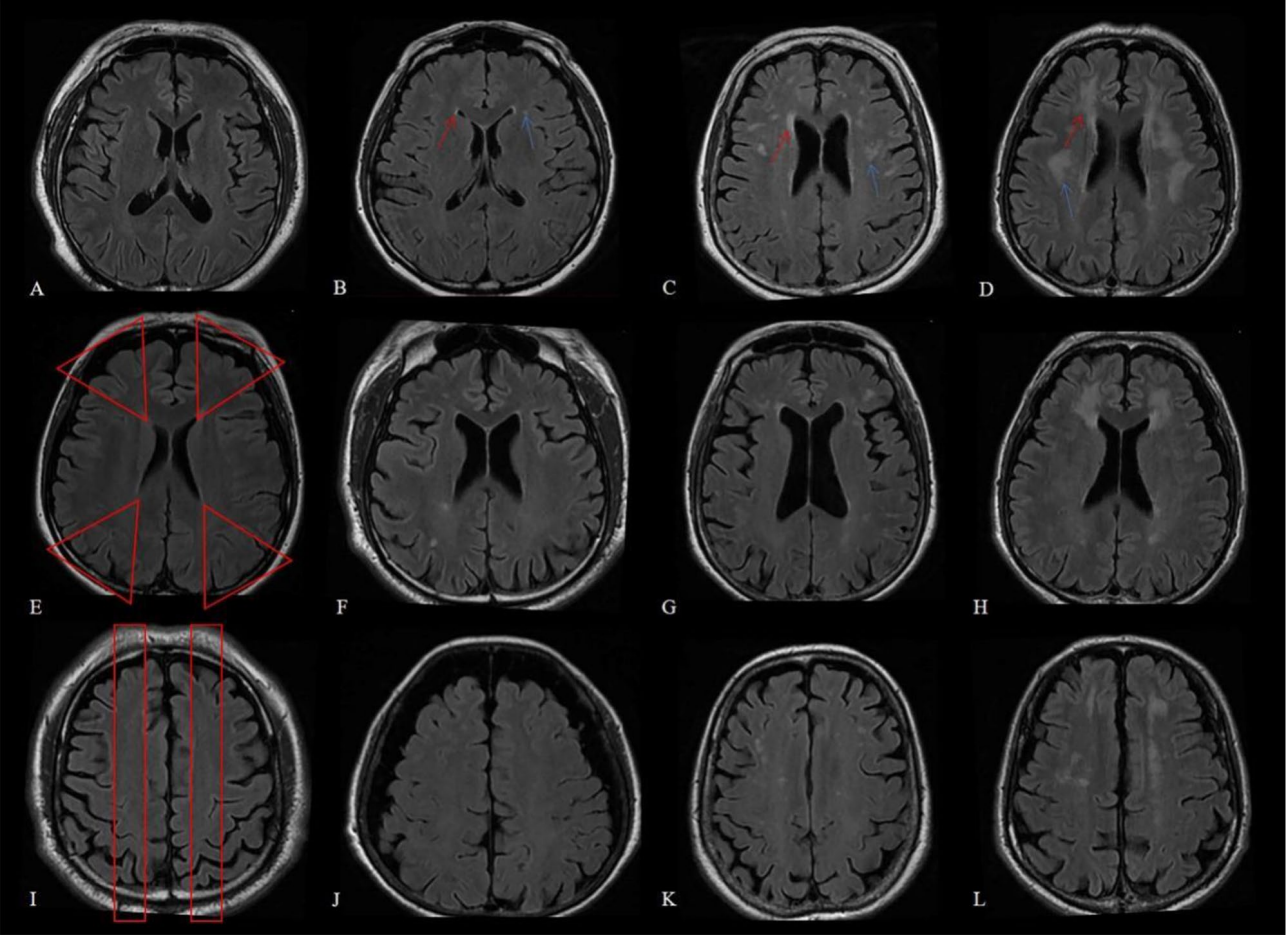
Examples of different categories of WMH scoring from 0 to 3 in the periventricular and deep white matter areas and CW area. FLAIR (A-D) showed the WMH in the periventricular (red arrow) and deep white matter areas (blue arrow), and from left to right, the scores went from 0 to 3 by the Fazekas visual rating scale [21, 22]. FLAIR (E-H) showed the WMH in the lower-level CW area (marked red), and from left to right, the scores went from 0 to 3. FLAIR (I-L) showed the WMH in the up-level CW area (marked red), and from left to right, the scores went from 0 to 3. WMH = white matter hyperintensities; CW = cerebral watershed
